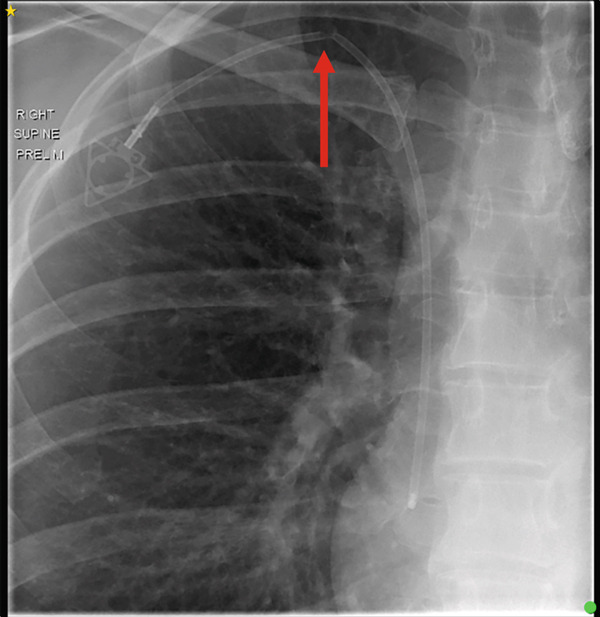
Chest radiograph demonstrating an incomplete fracture of the portacath at the internal jugular vein entry site (arrow).
